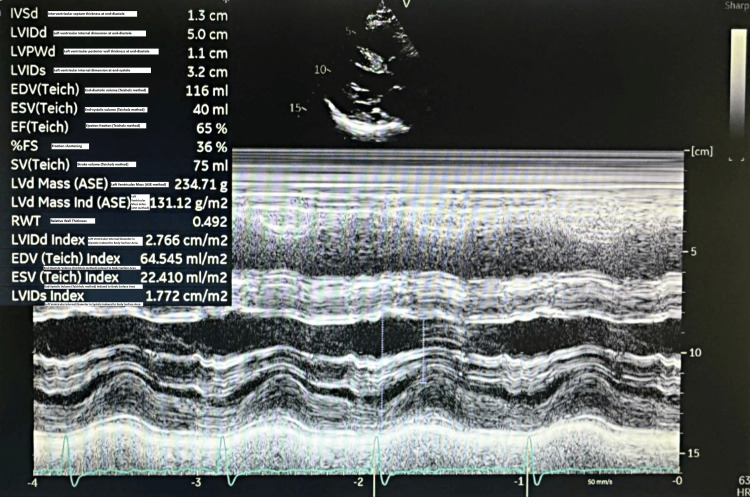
Transthoracic echocardiogram visualizing the normal left ventricular function and structure.
